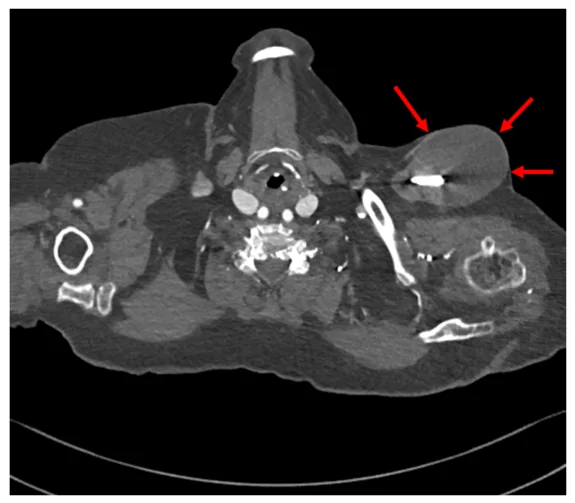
Thoracic computed tomography (CT) angiography showing a voluminous, thin-walled cystic lesion of liquid density (11 × 5 × 6.8 cm; 200 mL volume) encapsulating the pacemaker and proximal leads (red arrows).
